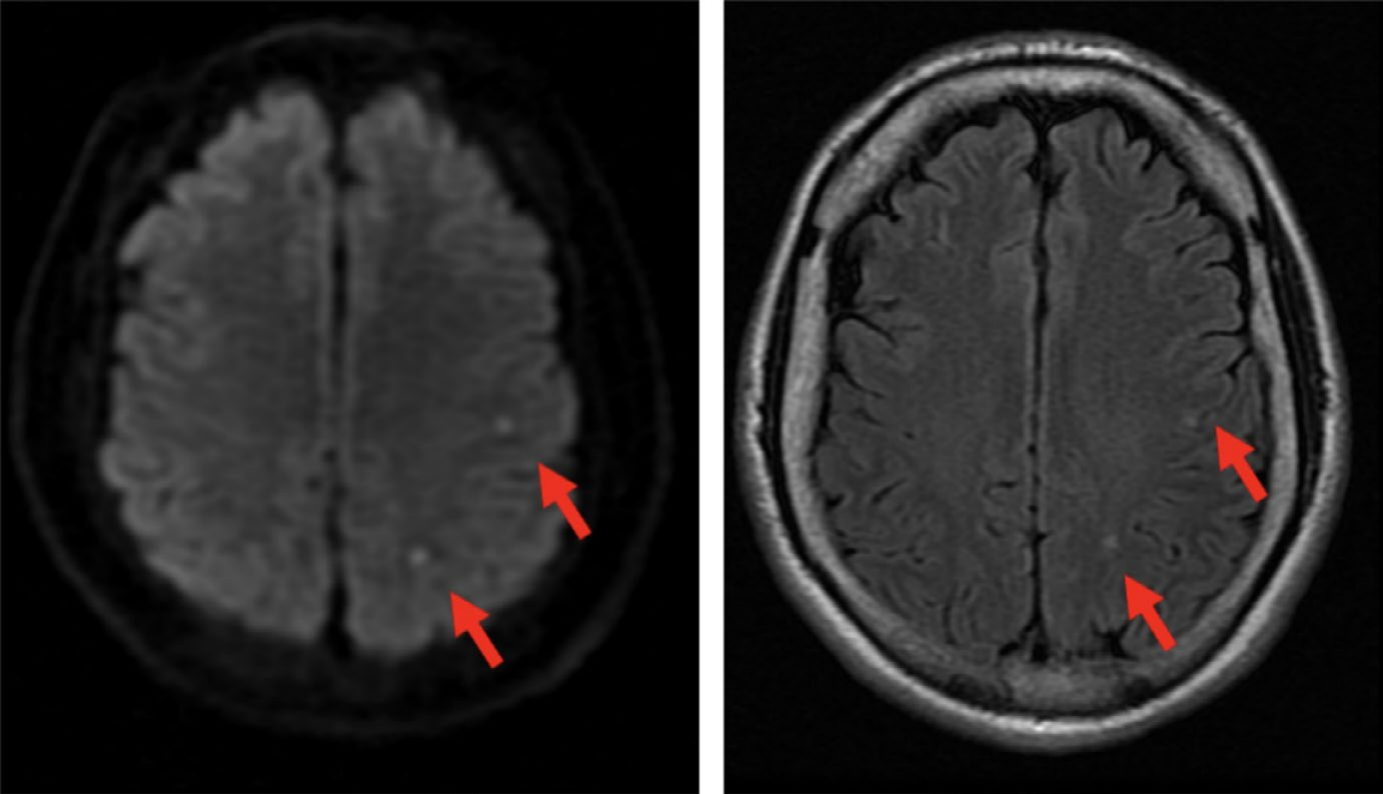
MRI DWI and Flair sequence showing two tiny infarcts within the territory of posterior left MCA
